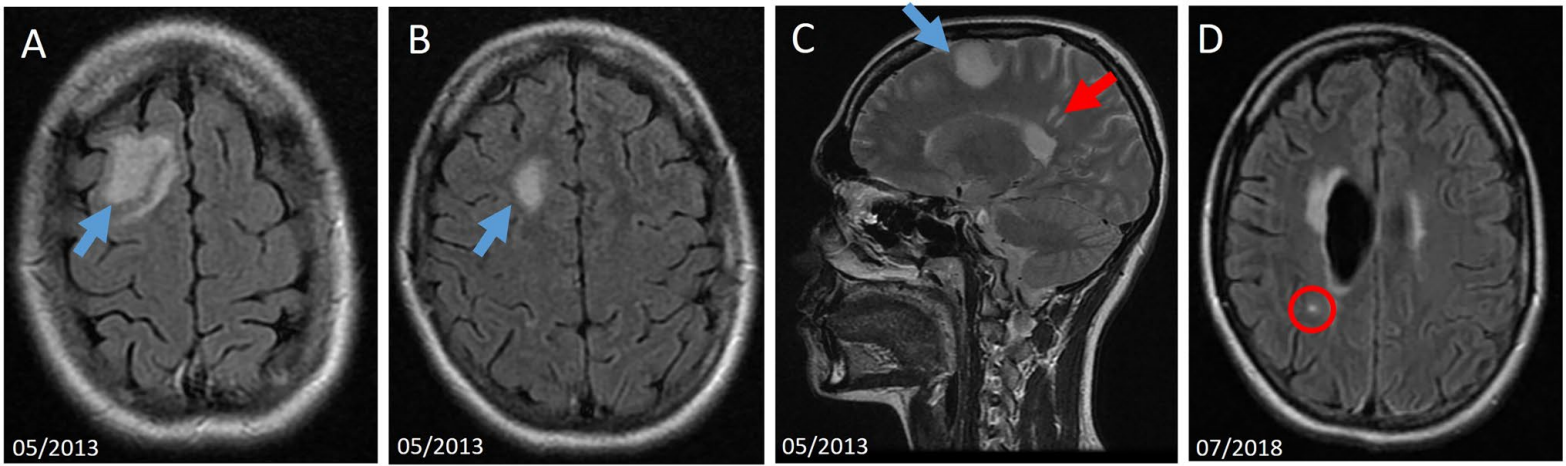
Resuming DMT in concomitant MS and CNS tumor. A–C This 21-year-old female patient presented for a second opinion at our hospital. She had been diagnosed 4 years before that with a relapsing–remitting multiple sclerosis (MS). During the diagnostic process, a right frontal lesion not typical for MS was detected (indicated by blue arrows; the red arrow shows two exemplary MS lesions). During the last follow-ups, the right frontal lesion has been expanding slowly. Therefore, primary resection was recommended and done 14 months after the first presentation in our clinic, leading to the diagnosis of anaplastic astrocytoma and followed by radio- and chemotherapy. D Four years later, the patient presented with a new numbness of the right arm. There were no signs of a tumor recurrence. A new right hemispheric T2/FLAIR-hyperintense lesion was detected on the brain MRI (red circle). No spinal MRI was done. Based on the new clinical relapse and radiologic signs of activity, the resumption of a disease-modifying therapy (DMT) for the MS, which had been stopped before the chemotherapy, was recommended
